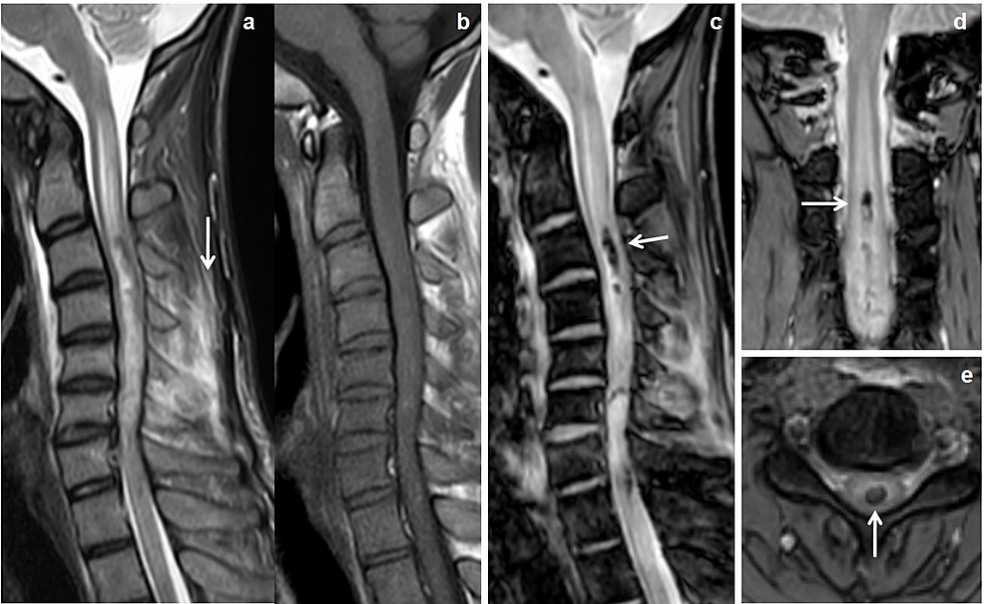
Cervical cord hemorrhage. Day 2 MRI scan of a 30-year-old male patient with history of RTA had quadriplegia. Sagittal STIR and T1W images (a & b) showed T2 hyperintense long segment cervical cord edema extends from cervico-medullary junction to C7 vertebral level with STIR hyperintensities in lower cervical inter and supraspinous ligaments (↓arrow). Sagittal mFFE image (c) showed multi-level cervical cord hemorrhages (← arrow). Coronal and axial mFFE images (d & e) showed central cervical cord hemorrhages (→ & ↑ arrows). STIR: short tau inversion recovery; mFFE: multiecho fast field echo; RTA: road traffic accident.
